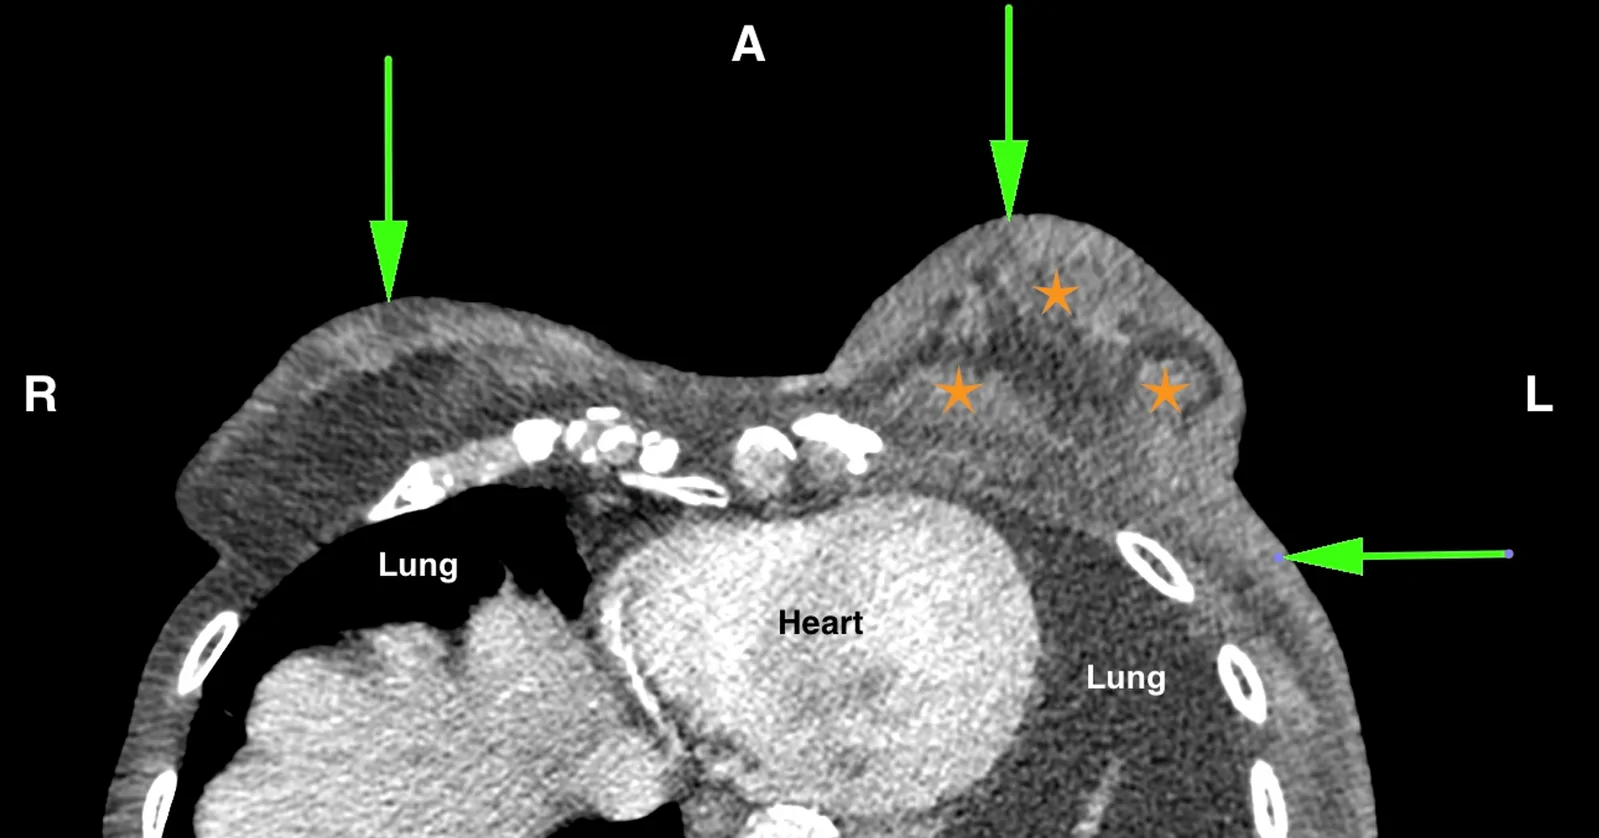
Labelled contrast-enhanced CT scan images (partial axial view) demonstrating irregular skin thickening of the bilateral breasts and anterior chest wall (green arrows). The soft-tissue densities are seen within the left breast (orange star). Also, note a left pleural effusion. The heart and lungs are labelled for reference. R, patient's right; L, patient's left; A, anterior of the patient; CT, computed tomography
